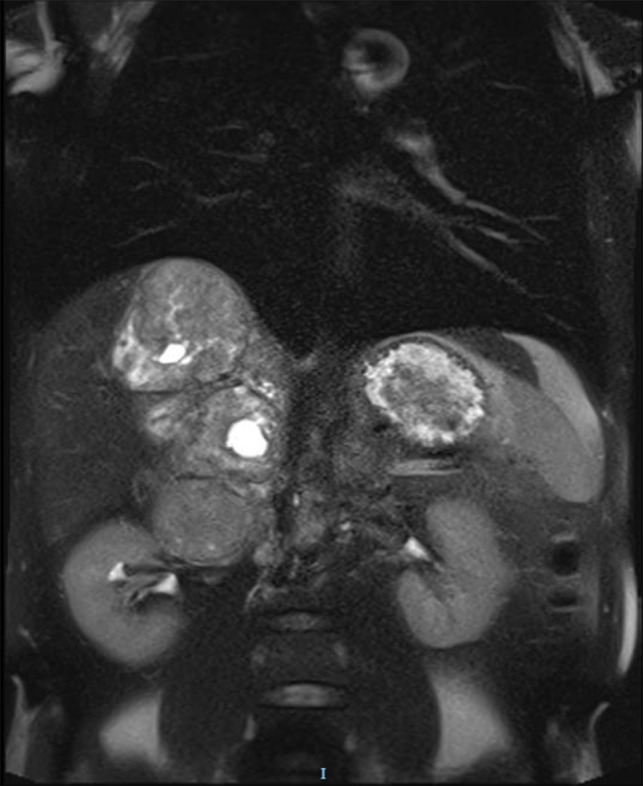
T2-weighted MRI coronal plane.
